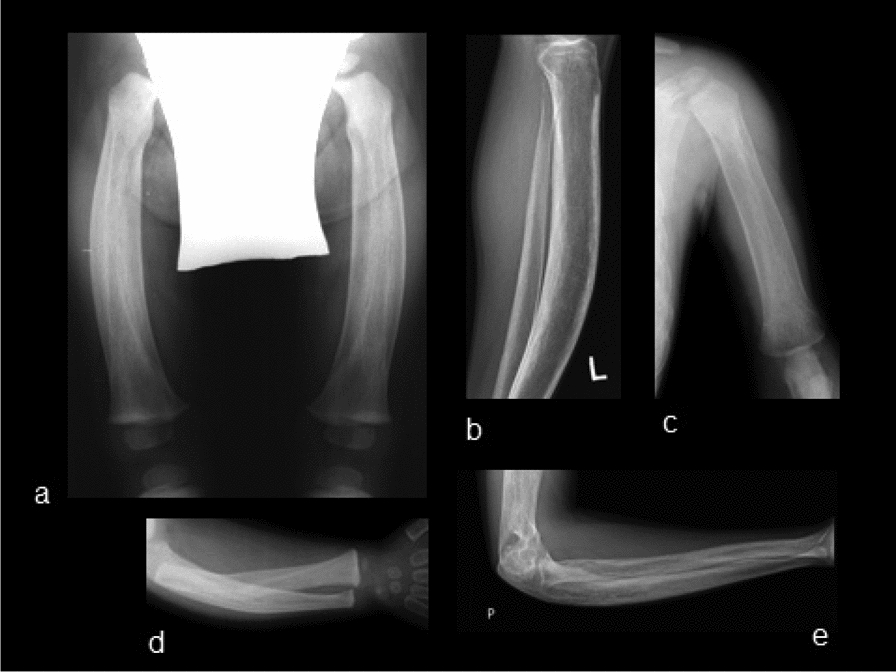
Radiography of long bones (a both femurs anteroposterior (AP), age 1 year (Y); b left tibia lateral, age 22 Y; c left humerus AP, age 1 Y; d right forearm AP, age 1 Y; e: right forearm lateral, age 22 Y). All radiographs reveal a diffuse modeling disorder with diaphyseal and metaphyseal undertubulation due to an extremely thickened cortical bone composed of widespread smooth periosteal appositions (featuring a “bone in bone” image), with a various degree of deviation. Diffuse osteosclerosis and a lesser degree of trabecular coarsening can be seen. Epiphyses have been spared
